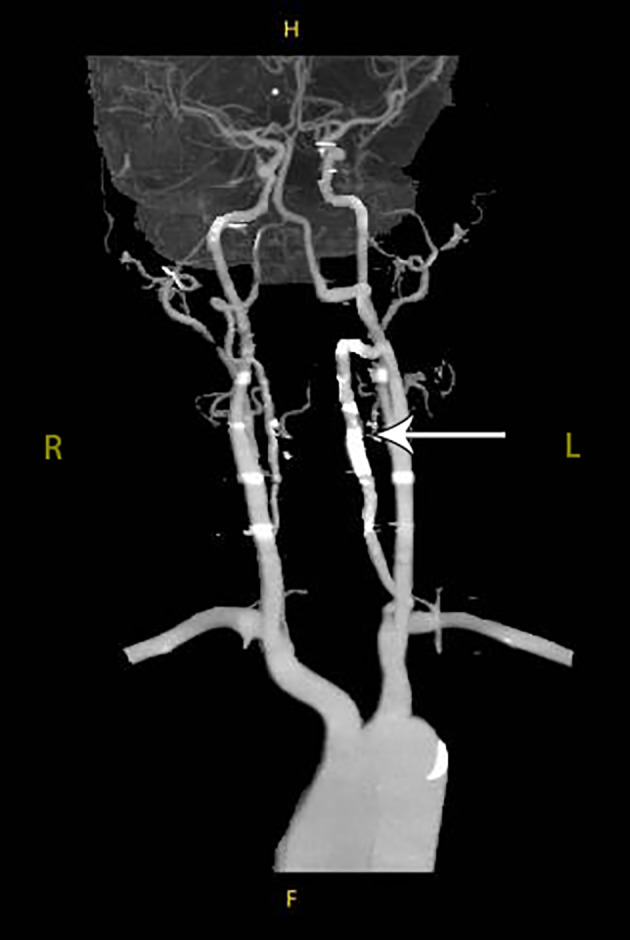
Three‐month follow‐up CTA of the vertebral artery showing a similar diameter of the lumen of the left vertebral artery with good flow compared to the preoperative status (arrow), with no residual pseudoaneurysm formation. CTA, CT angiography
